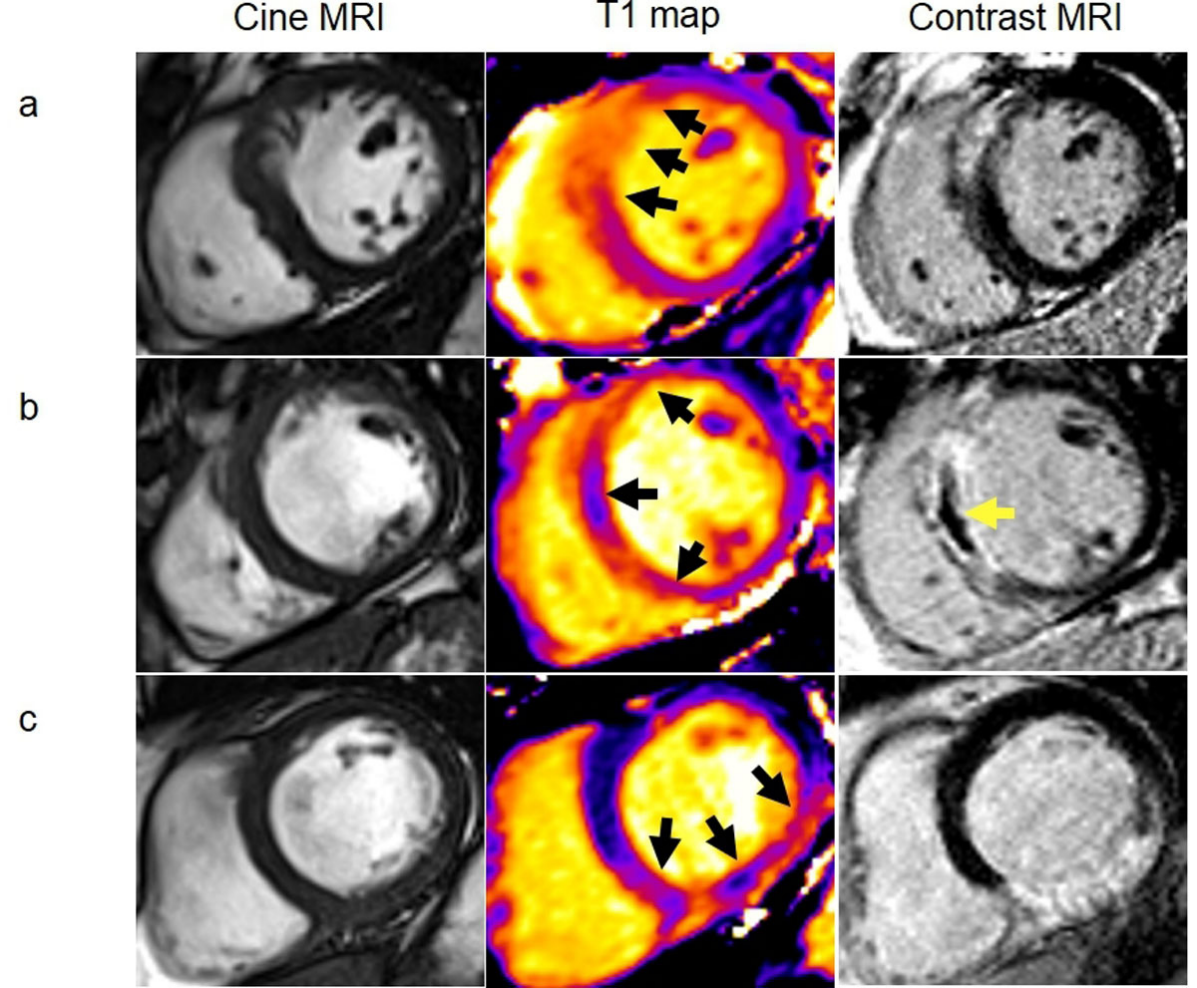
Three patients with acute STEMI treated by primary PCI. Cardiac MRI was performed for each patient 2 days later. (a) *Patient with no T1 hypointense infarct core and no microvascular obstruction.* Six month follow-up MRI revealed final infarct size was 15.6% of left ventricular mass and left ventricular end-diastolic volume (LVEDV) reduced was reduced vs. baseline scan. This patient had an uncomplicated clinical course. (b) *Patient with both T1 hypointense infarct core and microvascular obstruction.* Six month follow-up MRI revealed final infarct size was 22.6% of left ventricular mass and an increased LVEDV. During follow-up this patient was hospitalized for new onset heart failure. (c) *Patient with T1 hypointense infarct core*, *but no microvascular obstruction.* Six month follow-up MRI revealed final infarct size was 22.1% of left ventricular mass and a significantly increased LVEDV. No adverse events during follow-up.
